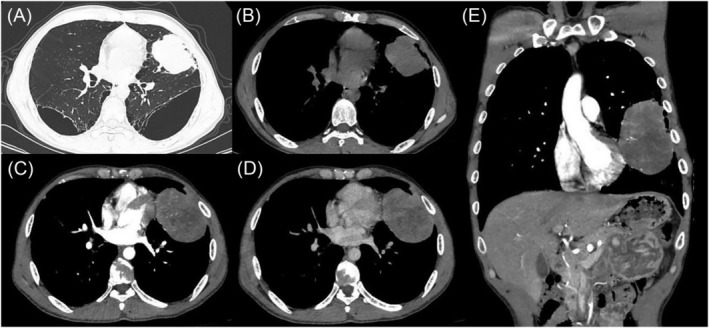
(A,B) Non‐contrast chest computed tomography (CT) scan revealed a soft tissue in the left upper lobe, measuring 47 × 39 mm in size. (C–E) Three months later, contrast‐enhanced CT showing the mass appeared inhomogeneous enhancement, which was larger than before, measuring 91 × 61 mm in size.
